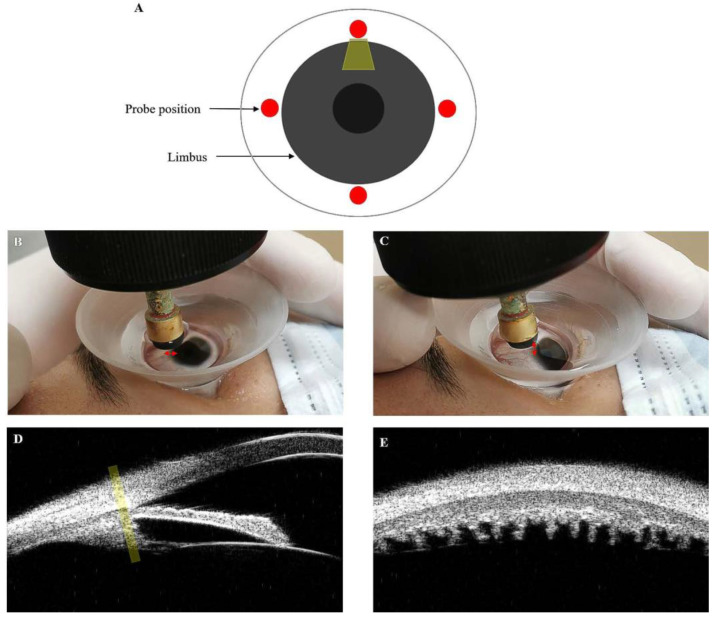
UBM was used to obtain the images of the ciliary body. The ultrasound beam appears in yellow. (A) Enface view with the probe position at 9, 12, 3, and 6 o’clock perpendicular to the limbus in red. (B,C) These images show the position of probes and eyes when obtaining superior radial and transverse UBM images, respectively. The red arrow shows the direction in which the probe swings during the examination. (D) This UBM image shows the relationship of the ultrasound beam to the CB when the transverse scan is performed. (E) This image depicts a transverse (superior) UBM image.
